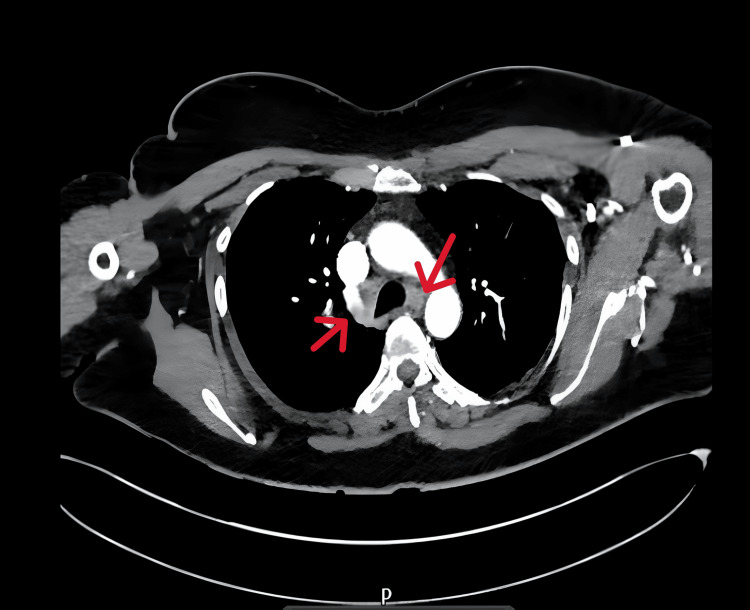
Sagittal CT of the chest showing bilateral mediastinal lymphadenopathy A key differential diagnosis for this radiological finding is sarcoidosis.
